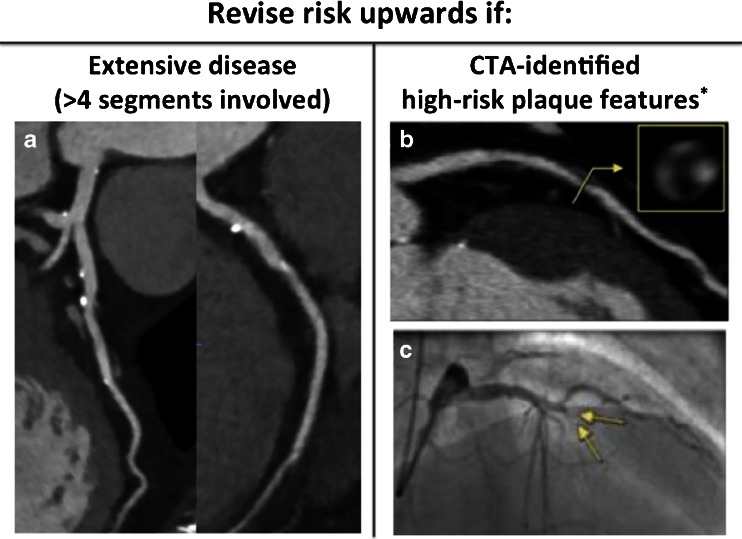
Coronary CTA features associated with an increased risk of major adverse cardiac events. a Extensive nonobstructive calcified plaque in the left main, proximal-mid LAD, proximal left circumflex, and first obtuse marginal arteries. b An atherosclerotic plaque with positive remodeling, low-attenuation plaque, and napkin-ring sign in the proximal LAD on coronary CTA*. c Invasive coronary angiography demonstrating occlusion of the proximal LAD at the site of high-risk plaque 10 months after coronary CTA (*arrows*). *Reproduced with permission from Otsuka et al. [41]. *CTA*, computed tomographic angiography; *LAD*, left anterior descending
